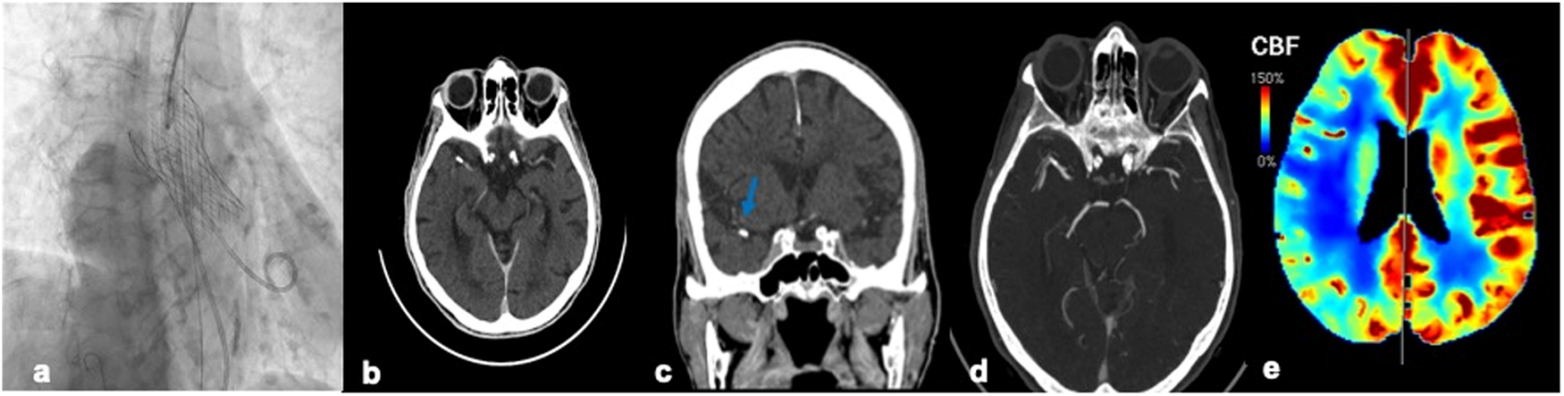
An 83-year-old patient presenting to the emergency room with dysarthria, difficulty in walking, and dropping lip 7 days after TAVI for severely calcific aortic-stenosis (a). A brain CTA was performed, demonstrating a calcific embolus in the right middle cerebral artery (b, c arrow), determining incomplete occlusion of the vessel (d, arrow), as a result of distant calcific embolization during TAVI. Perfusion-CT shows an area of ischemic penumbra in the right cerebral hemisphere, with reduced cerebral blood flow (CBF) (e). The patient was treated with a thrombectomy and got a resolution of clinical symptoms
